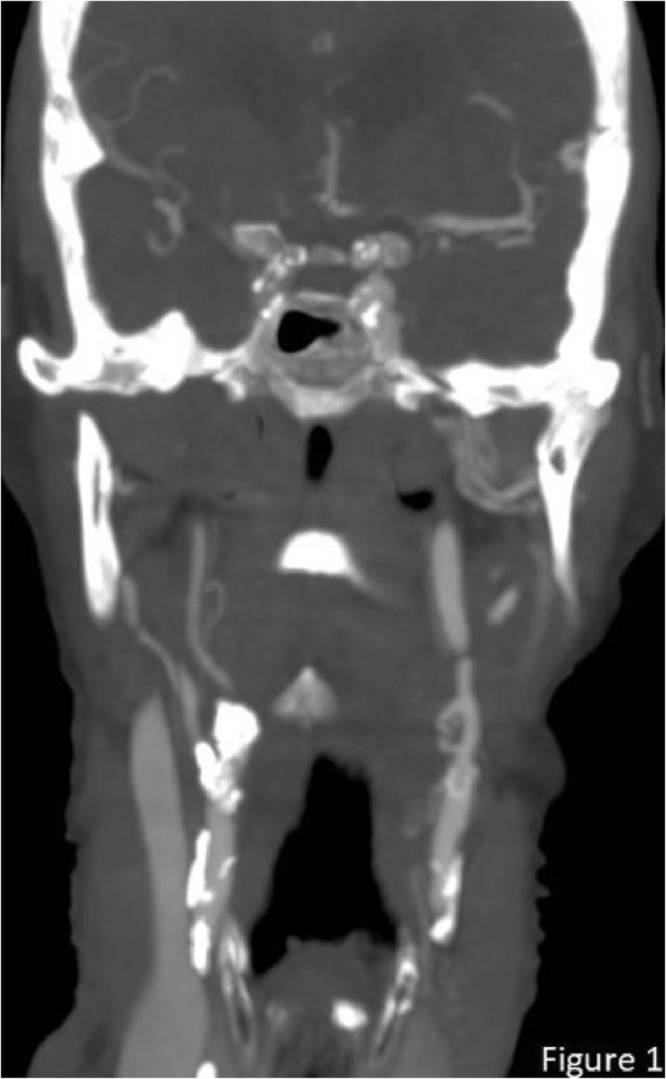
CT angiography coronal plan view showing a significant stenosis in the right internal carotid artery and the passage of contrast distal to the stenotic segment.
